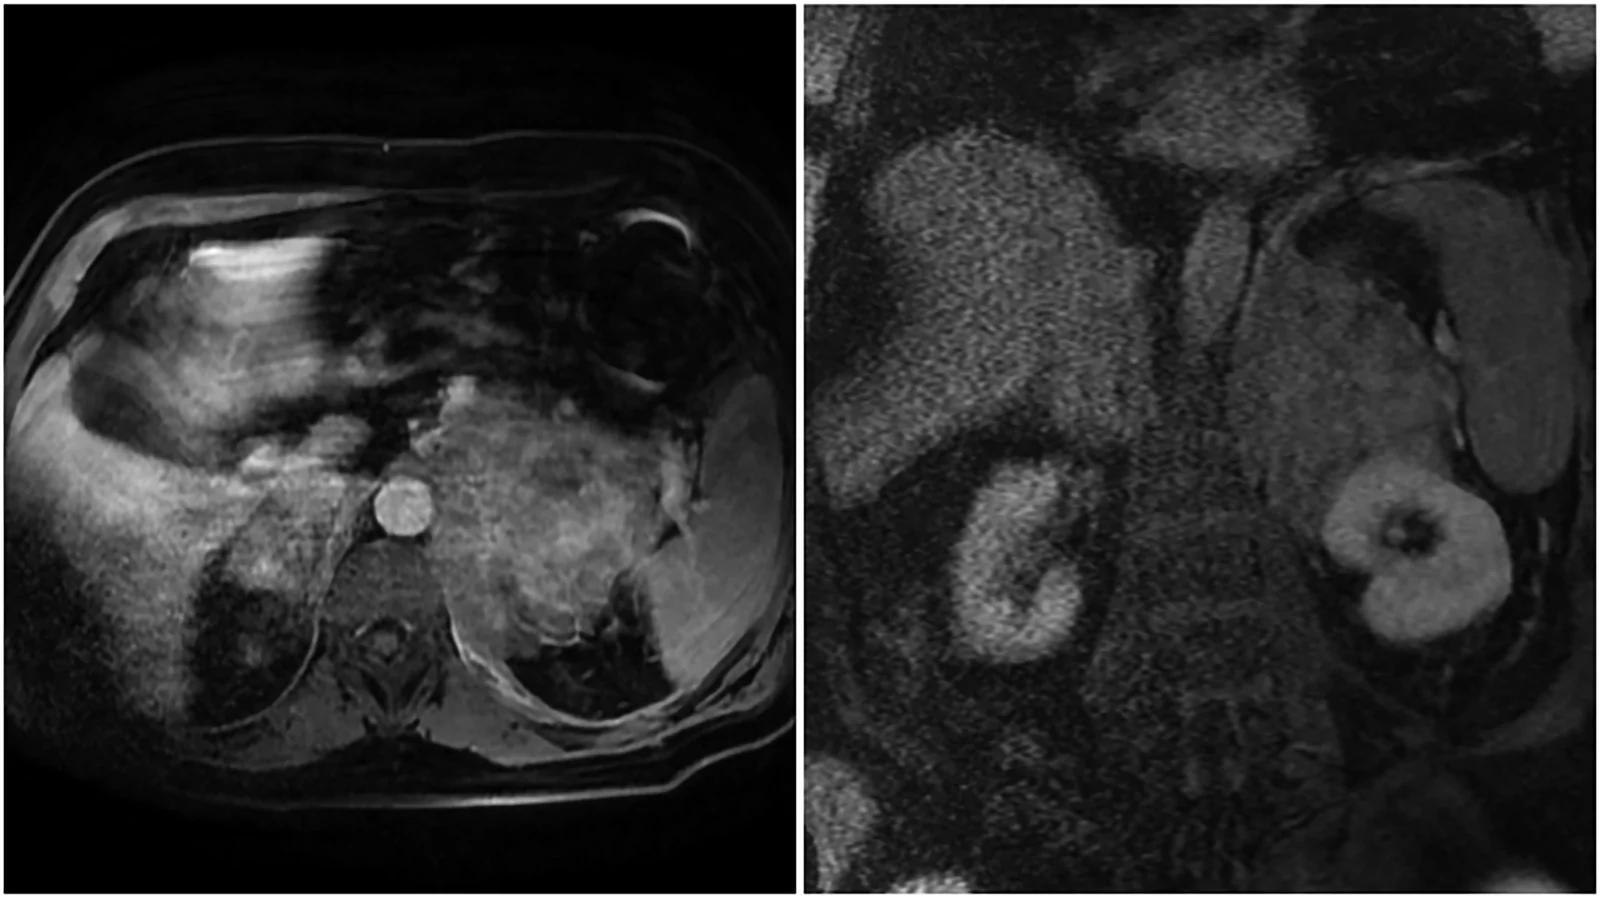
Post-contrast fat-suppressed T1-weighted axial and coronal MR images demonstrate bilateral adrenal masses with heterogeneous enhancement.
